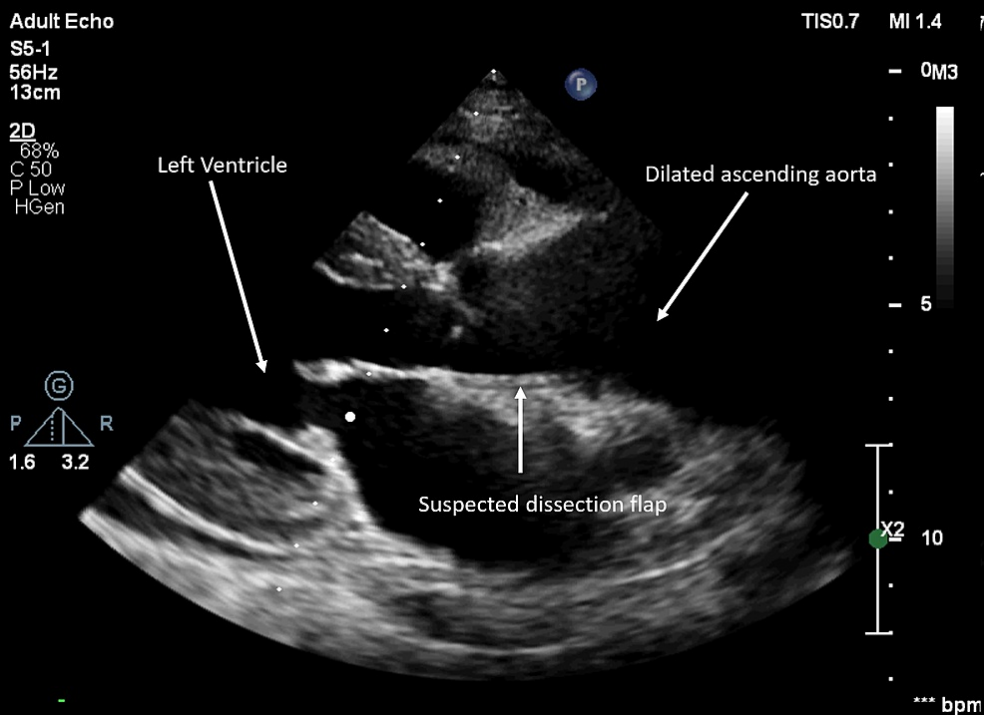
Parasternal long-axis view showing a dilated ascending aorta.
